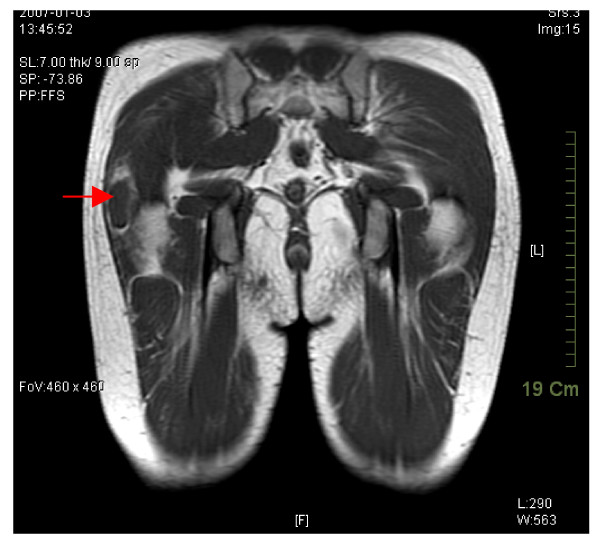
Magnetic resonance imaging study revealing a focal lesion within the lateral portion of the gluteus maximus muscle. Hypointense signal in T1W is seen under contrast enhancement.
